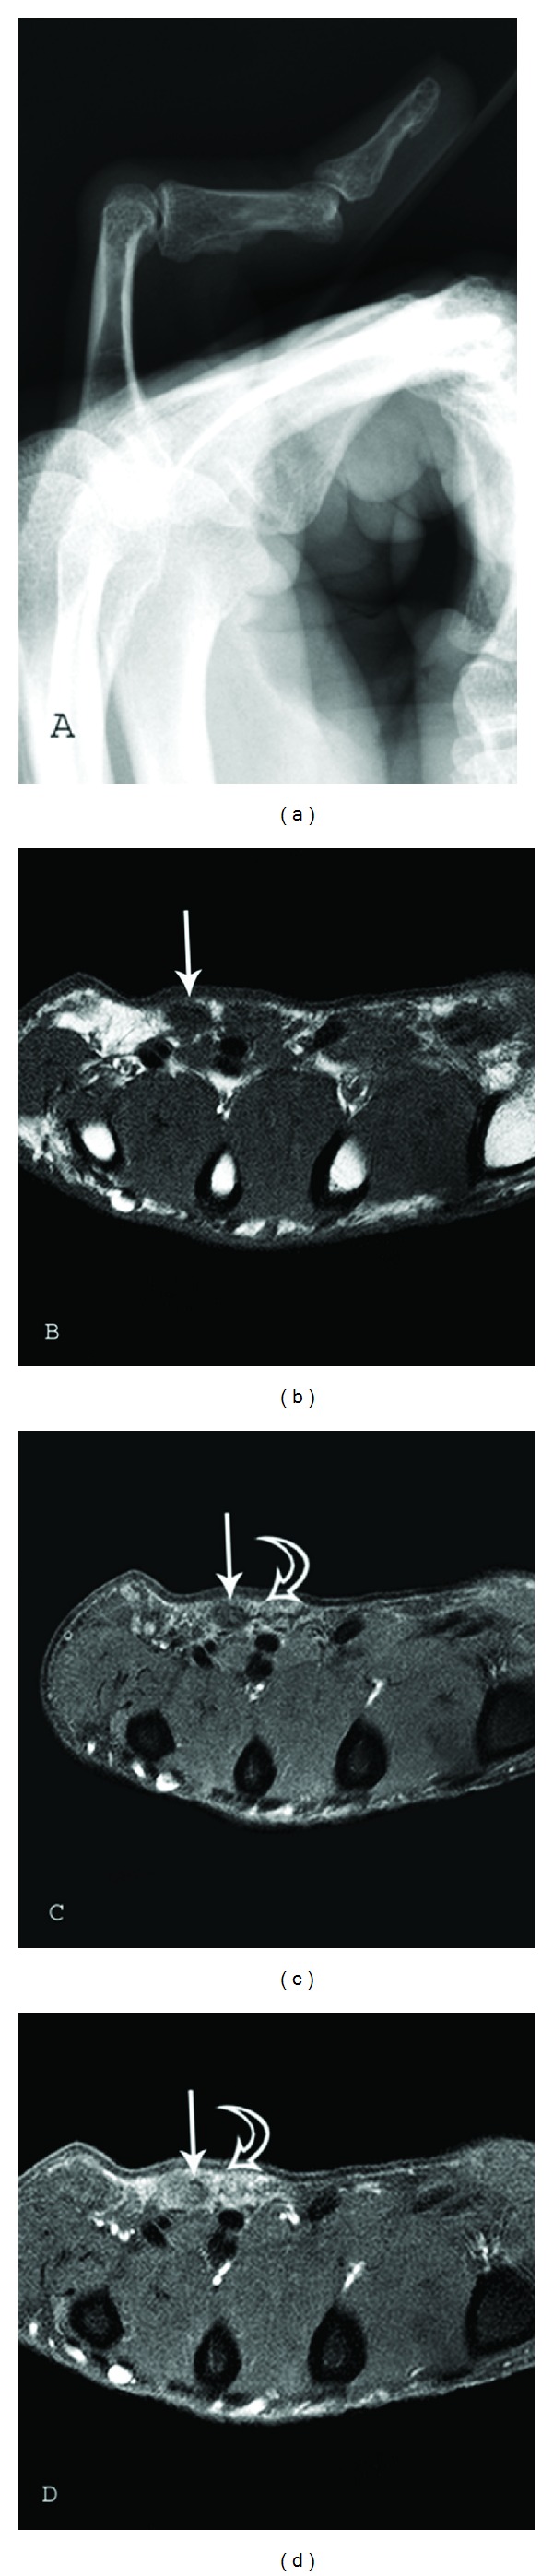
Palmar Fibromatosis. Lateral radiograph (a) of the right hand 5th finger in a 48-year-old man shows a flexion (Dupuytren) contracture of the proximal interphalangeal (PIP) joint. MR images of a 73-year-old man with pathologically proven palmar fibromatosis. (b) Axial T1-weighted (TR 500/TE 21) and (c) axial proton-density-weighted (TR 1500/TE 35) fat suppressed images of the hand at the level of the metacarpal bones show nodular areas of low-signal intensity in the volar subcutaneous fat (arrows) located superficial to the flexor tendons of the fourth and fifth fingers. Increased signal surrounds the nodules (curved arrow) on fluid sensitive sequence. (d) Axial T1-weighted (TR 500/TE 21) fat-suppressed image after administration of gadolinium contrast demonstrates moderate and diffuse enhancement (curved arrow) surrounding the nodules.
